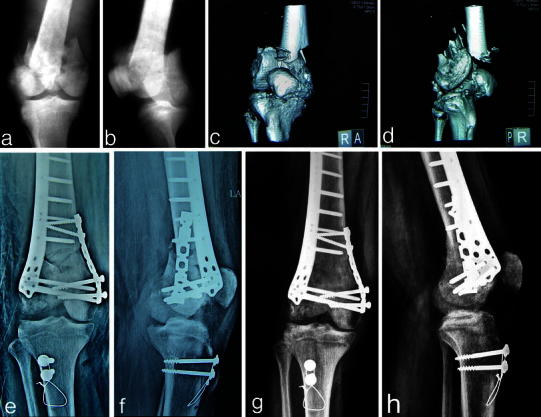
a, b Case number 12: AP and lateral plain X-ray views showing typical C3-type distal right femur fracture. c, d 3-D reconstruction, AP and lateral CT scans. e, f Immediate postoperative views showing the lateral locked condylar plate and the medial contoured reconstruction plate with its distal 6.5-mm cancellous screws with prominent heads due to mandatory obliquity of the screws inside the bone. g, h The same views after 9 months, showing complete radiological healing of fracture in good anatomical position with incorporation of the metaphyseal autogenous bone grafts and excellent healing of the tibial tuberosity osteotomy without loosening of the implants. The medial prominent screw heads did not bother the patient or decelerate the early aggressive rehabilitation program. The patient returned fully to his previous work with painless 130° knee flexion and no extensor lag
